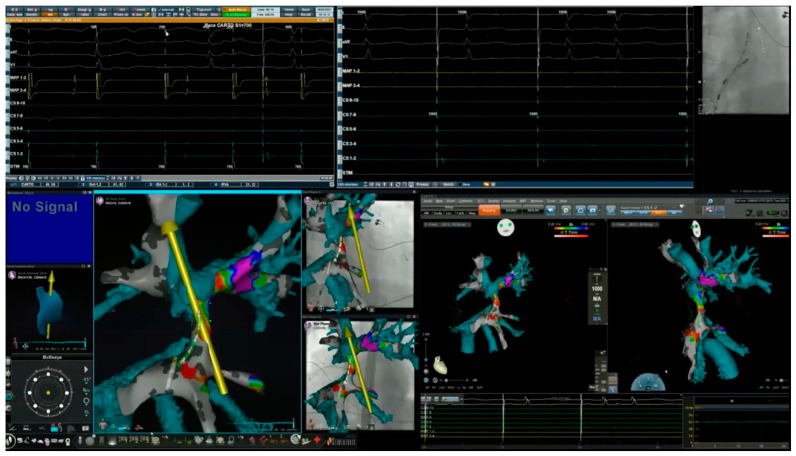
Merging of electroanatomic mapping and CT scan images of the systemic venous atrium in multiple views. Shown is a standard screen view from the control room during an ablation procedure, with the upper panels capturing snapshot (left) and real-time (right) electrogram tracings. The bottom panels display the operator view of CT scan and 3D mapping images (left) during the merging process, overlaying of these images on orthogonal fluoroscopic views (middle), and views from the 3D electroanatomic mapping system. The yellow arrows indicate the direction of the magnetic field.
